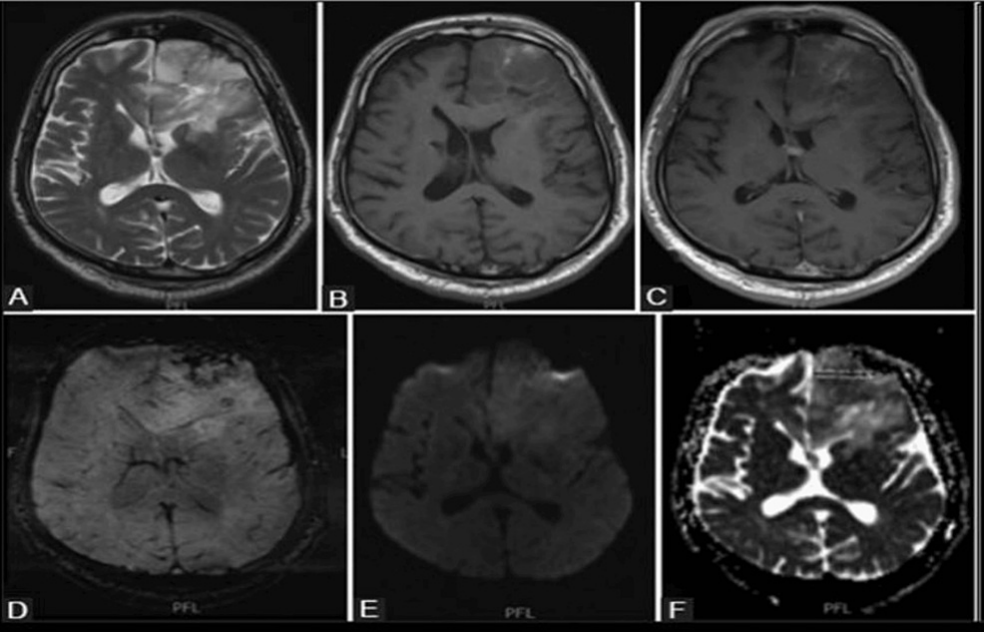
MRI of 1p/19q co-deleted low-grade glioma (oligodendroglioma). (A) Axial T2-weighted imaging (T2WI). T2WI is employed to visualize the anatomical structures with high water content. In the presented MRI, this sequence reveals the lesion in the left frontal lobe of the brain. (B) Axial T1-weighted imaging (TIWI). TIWI is utilized to highlight differences in tissue composition. The axial T1-weighted image in this figure provides additional details about the lesion's characteristics, helping in its assessment and classification. (C) Axial TIWI post-contrast administration). Post-contrast TIWI enhances the visibility of contrast-enhancing features, such as blood-brain barrier disruption. The axial post-contrast T1-weighted image in the figure allows for the identification of specific characteristics associated with the lesion, contributing to a more nuanced diagnosis. (D) Susceptibility-weighted imaging (SWI). SWI is sensitive to magnetic susceptibility differences between tissues, highlighting areas of blood or mineral deposition. The SWI sequence aids in detecting microhemorrhages or calcifications within the tumor, offering additional information for a comprehensive assessment. (E) Diffusion-weighted imaging (DWI). DWI assesses the movement of water molecules in tissues. In the presented MRI, the DWI sequence assists in evaluating the lesion's cellular density and organization. (F) Apparent diffusion coefficient (ADC) map. The ADC map is derived from DWI and provides quantitative information about the restriction of water diffusion within tissues. In Figure 2, the ADC map is utilized to calculate and visualize mean ADC values. A low mean ADC value is indicative of restricted diffusion, which can be associated with increased cellularity in the lesion.
